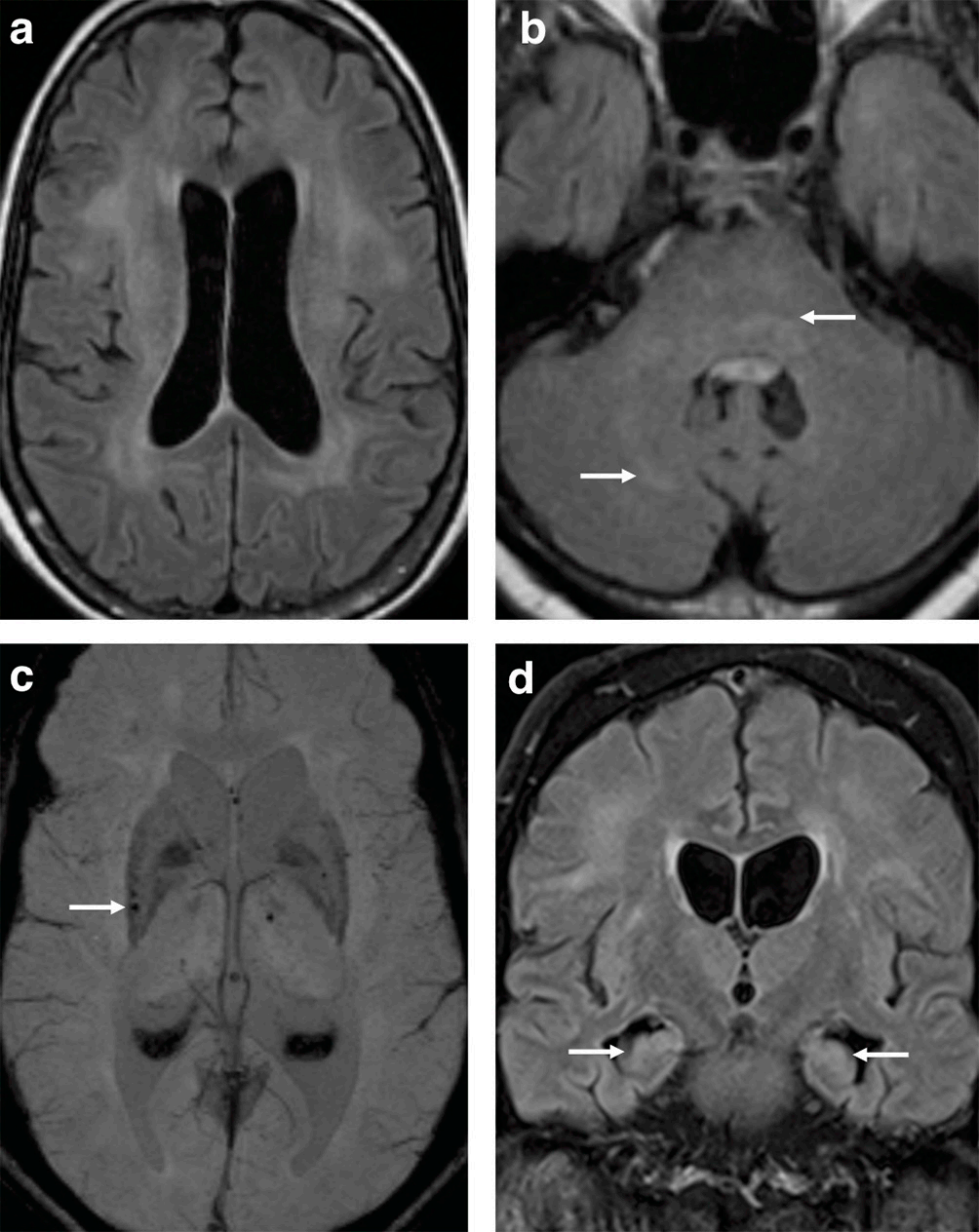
62-year-old male with West African Trypanosomiasis. (a,b) Axial T2W FLAIR demonstrating ventriculomegaly with bilateral deep white matter hyperintense signal extending from the corona radiata into the posterior limbs of the internal capsules (not shown) as well as the mid brain, pons and right cerebellum (arrows). MRI performed 3 months later shows new susceptibility artefact on SWI in the right posterior putamen (arrow) (c). (d) Coronal T2W FLAIR demonstrates some improvement in bilateral white matter changes and stable ventricular size with hyperintense signal in the hippocampi (arrows). SWI, susceptibility-weighted imaging; T2W FLAIR, *T*
